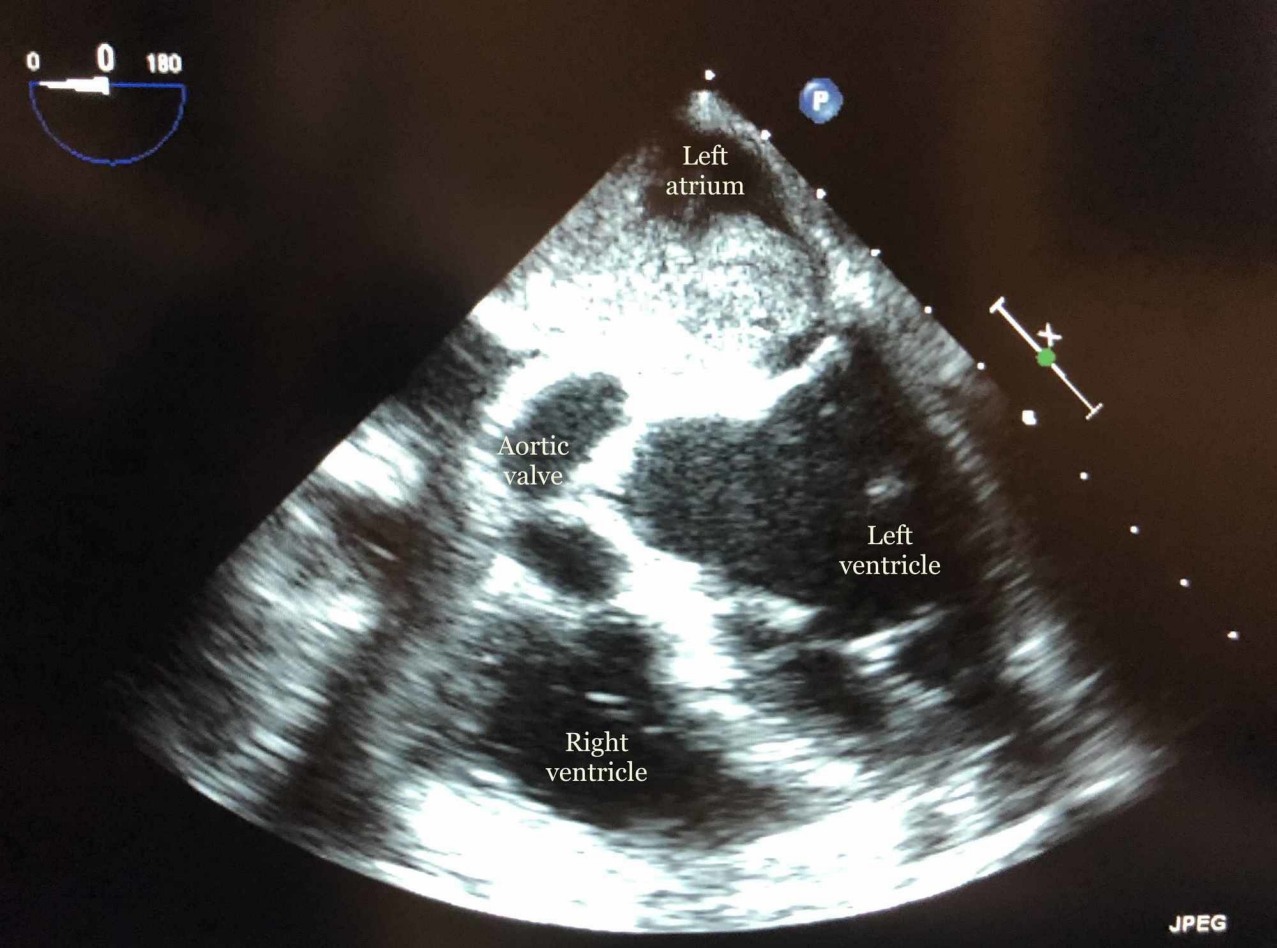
Areas of the heart visible from a transesophageal echocardiogram with myxoma in the left atrium.
